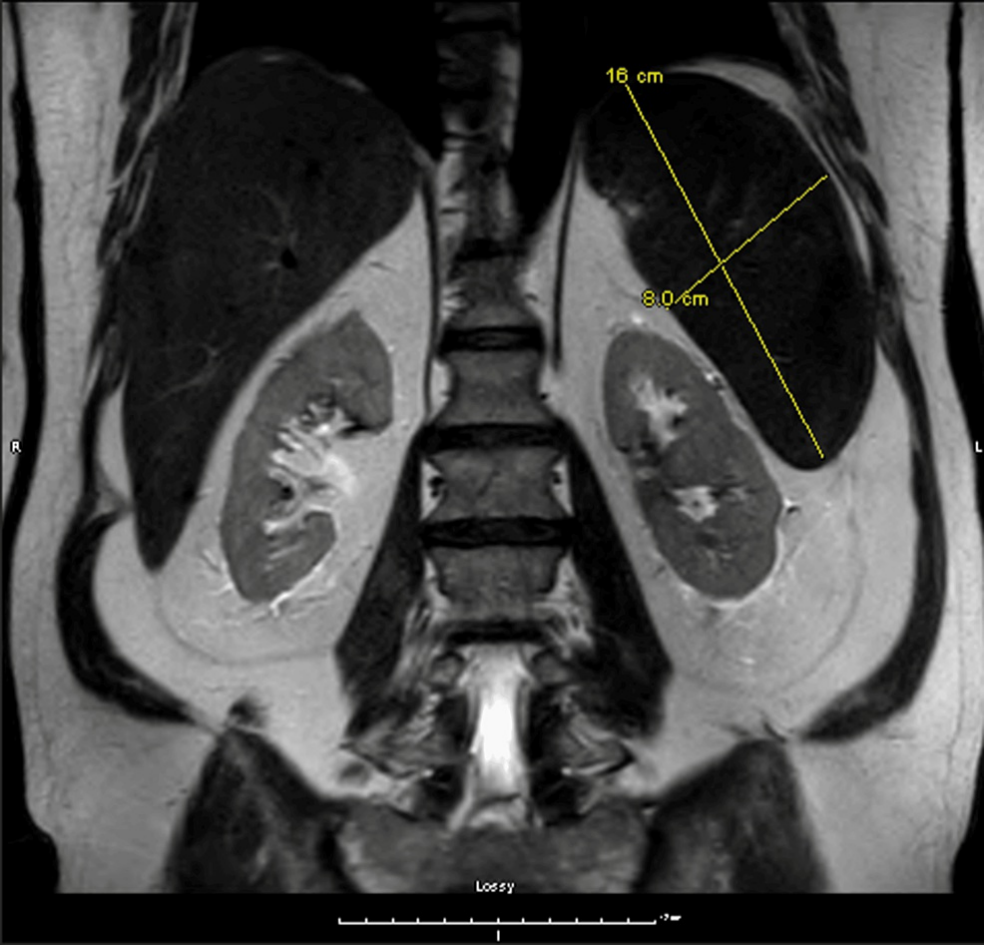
A T2-weighted TSC-corrected MRI image indicates splenomegaly and no identifiable renal mass.
